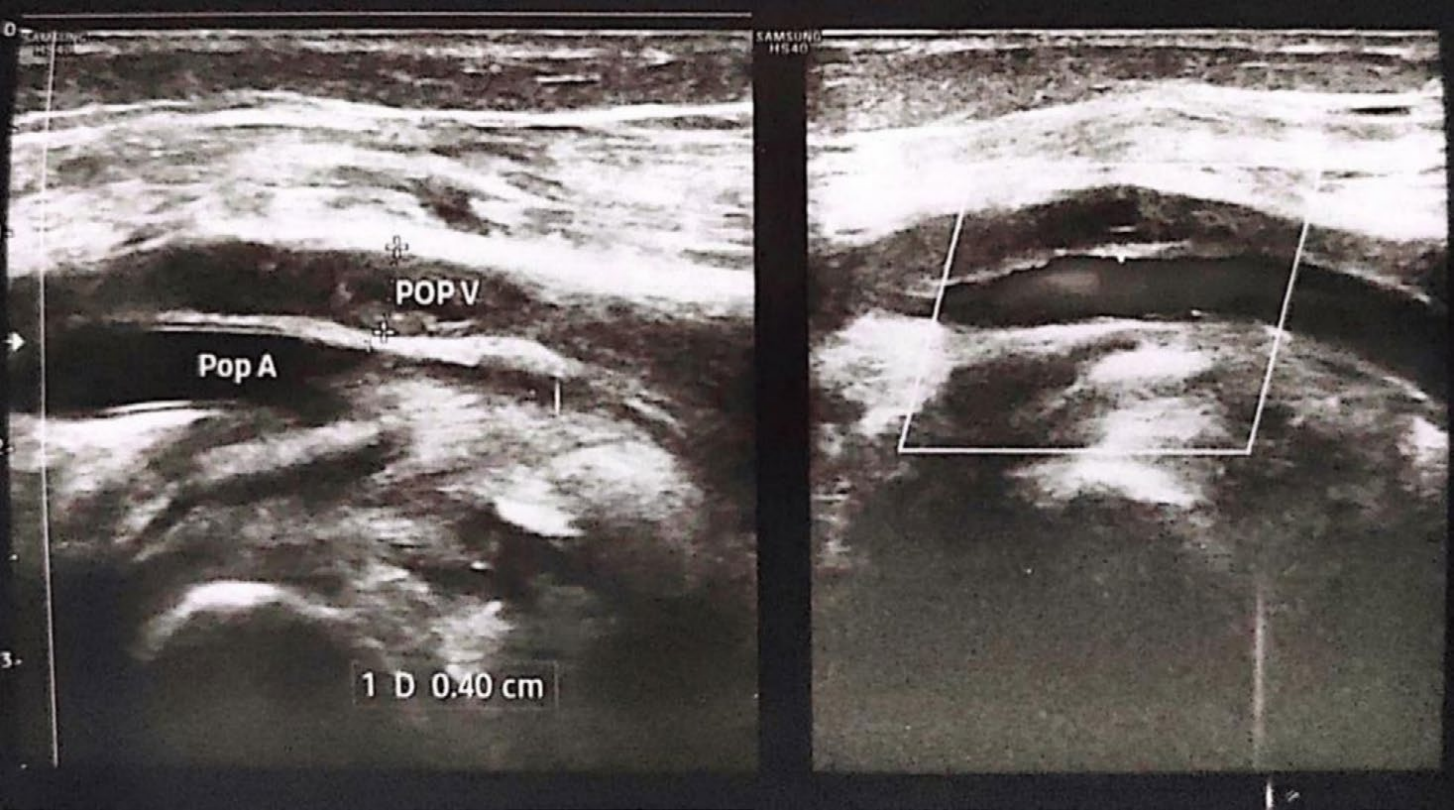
Duplex ultrasonography of the left lower limb showing echogenic thrombus causing luminal dilation and non‐compressibility of the popliteal vein, with complete occlusion of the common femoral vein and thrombus extension into the superficial femoral vein.
